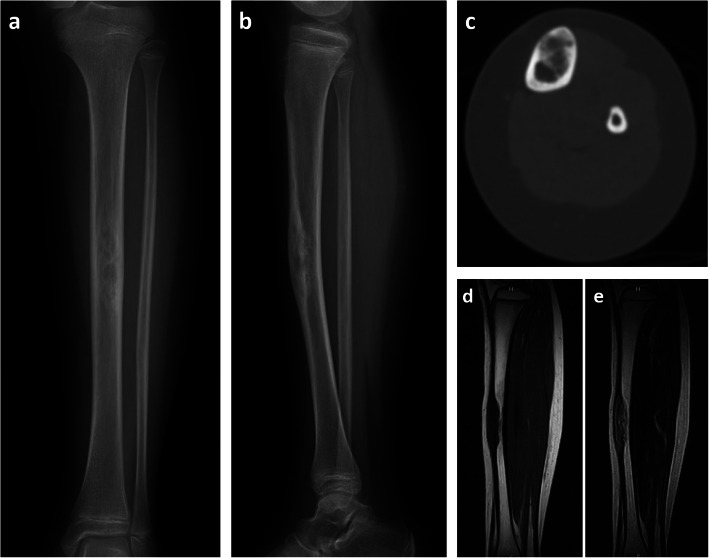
The X-ray, CT image, and MR images of the tumor. a Anteroposterior radiograph. b Lateral radiograph. c Axial CT image. d T1-weighted magnetic resonance sagittal image. e T2-weighted magnetic resonance sagittal image
